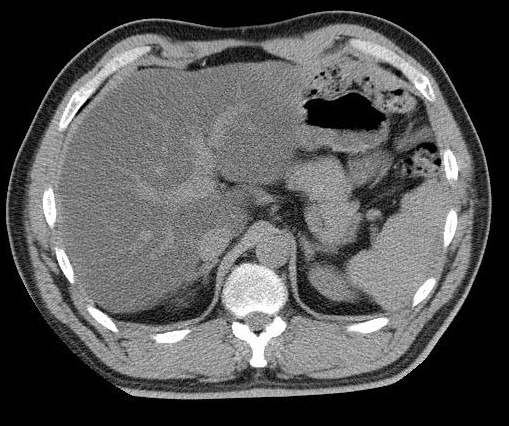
16 slices-unenhanced CT, which depicts lower attenuation values of liver tissue comparing with spleen
